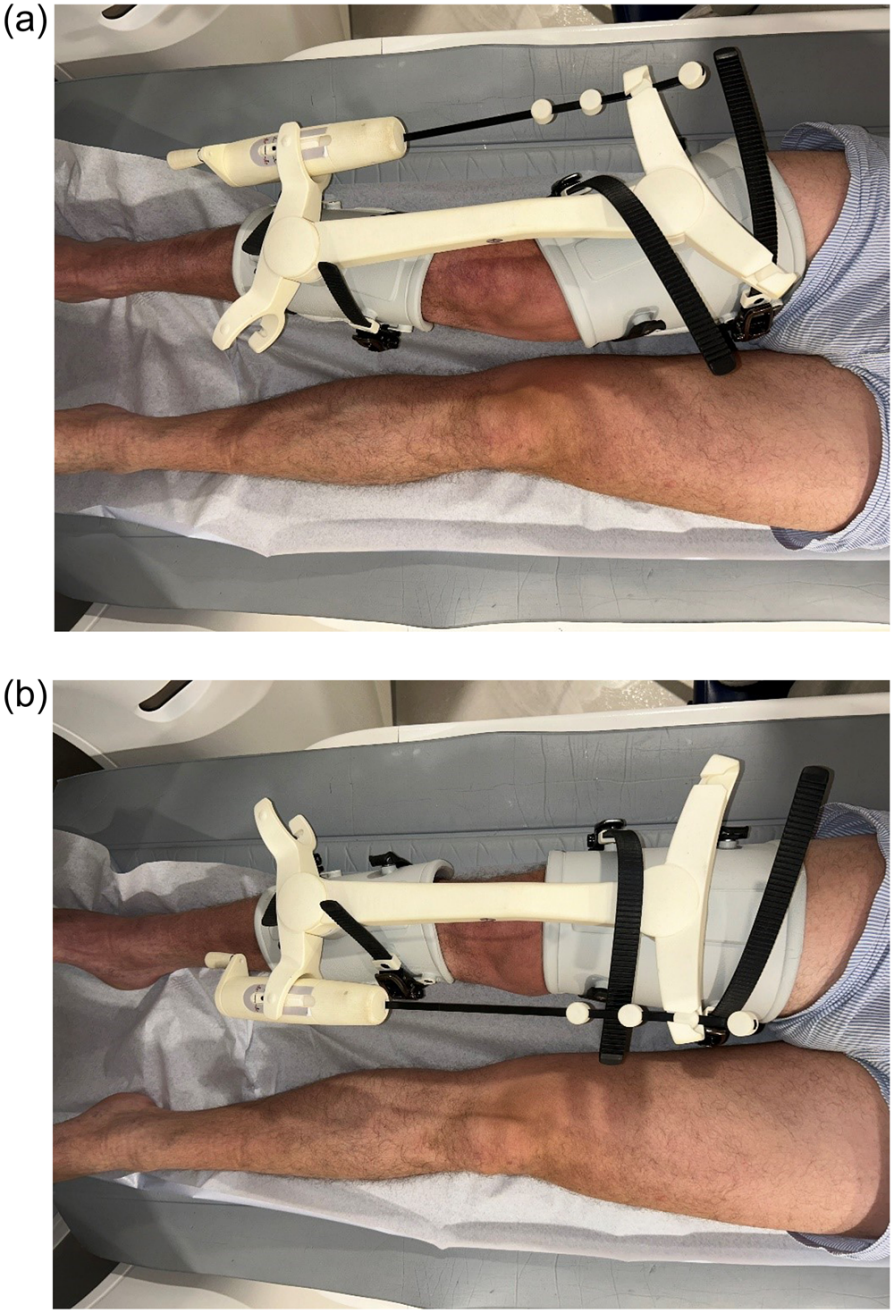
(a, b) Picture of the knee in CT scanner with the loading device in valgus and varus. CT, computed tomography.
